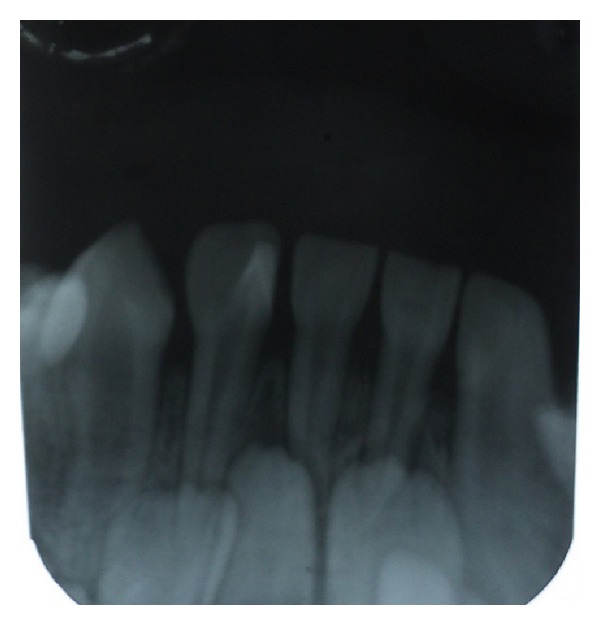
Radiographic image showing pulp extension into the talon cusp.
